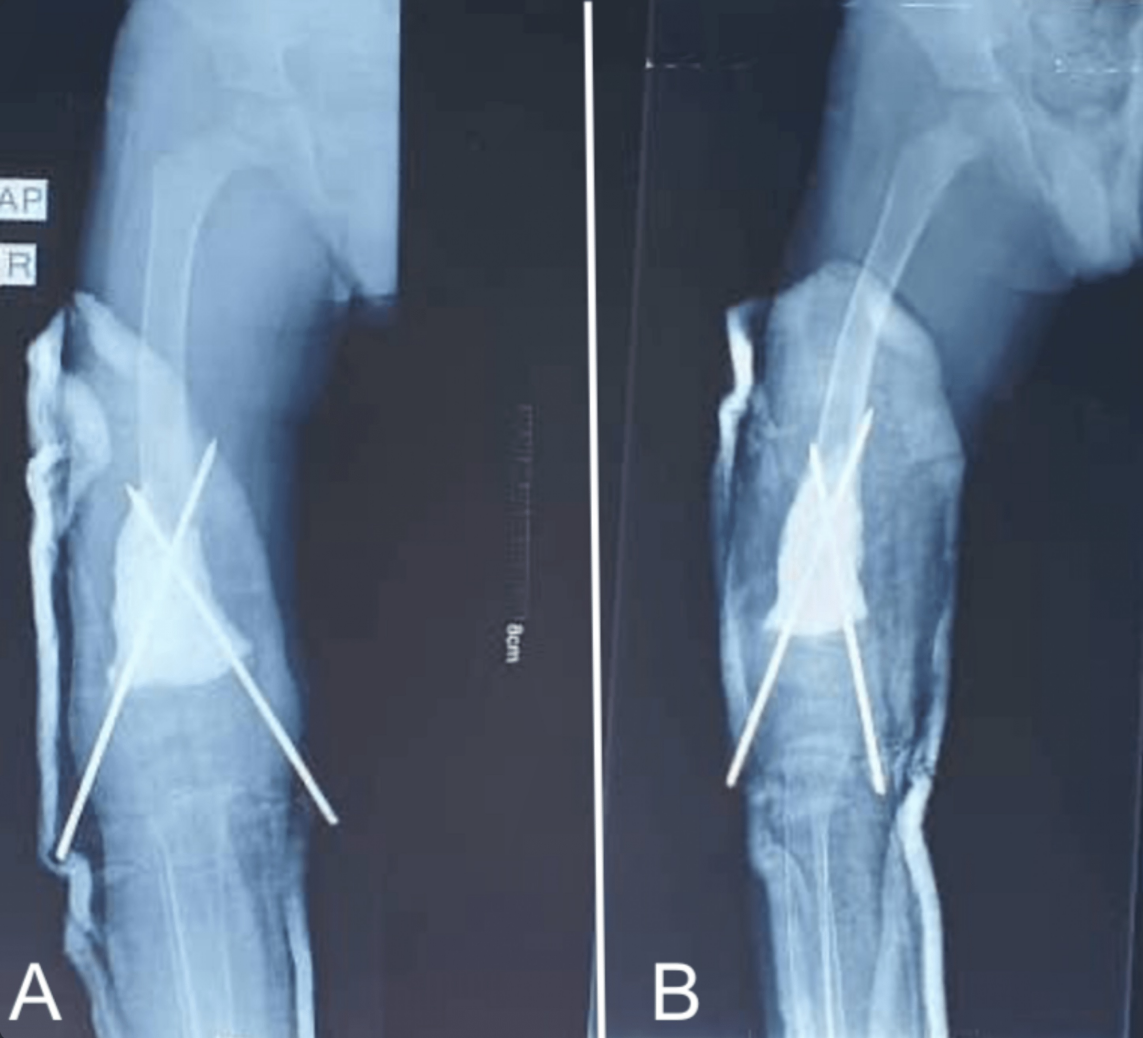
Postoperative X-ray following the first stage of the Masquelet technique. Bone cement placement with crossed pins for surgical fixation. The pin going to the medial side is too proud. (A) AP view; (B) Lateral view.
